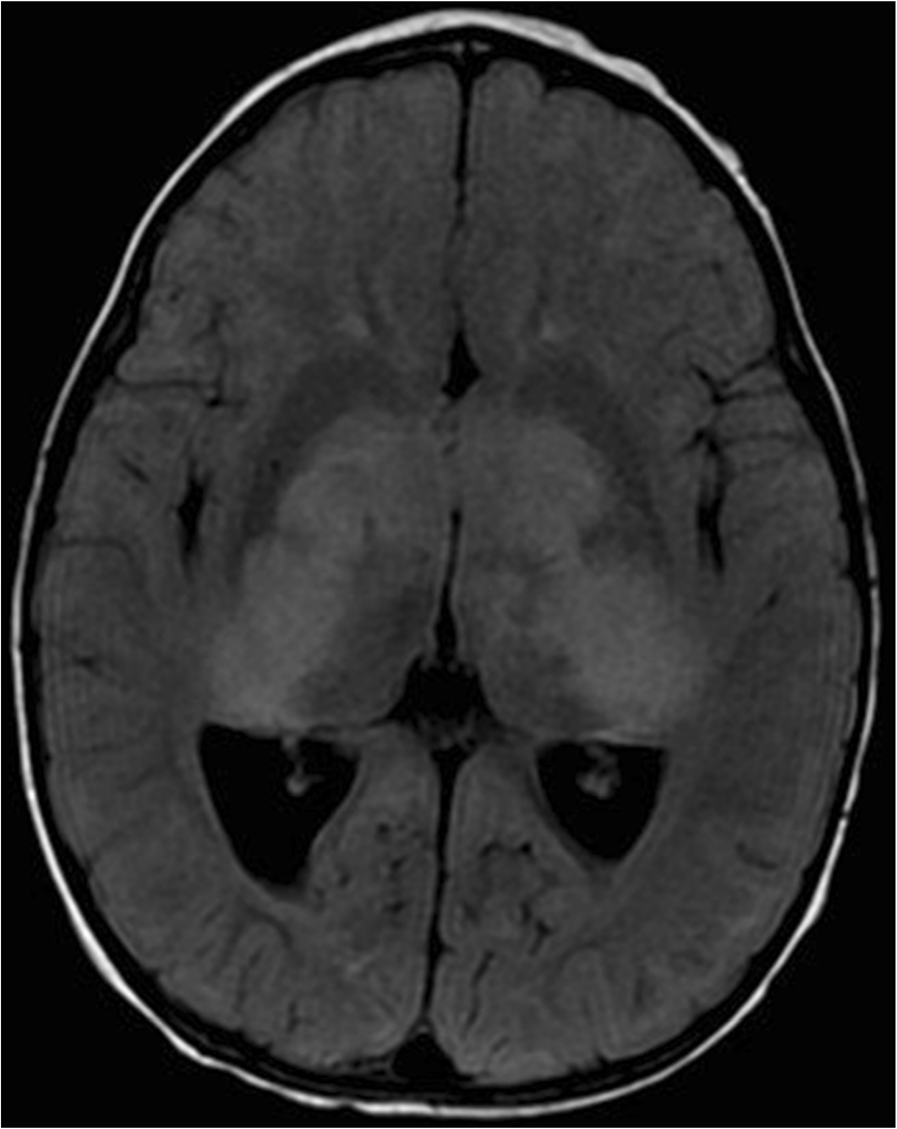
An axial brain MRI of a 22 months old female with NF1 is shown. There are bilateral extensive confluent areas of FLAIR signal abnormalities with increased signal intensity involving the internal capsule bilaterally, basal ganglia, thalami, hypothalamus, and cerebral peduncles. There is mass effect on the posterior horns of the lateral ventricles and on the third ventricle causing dilatation of the posterior horns
